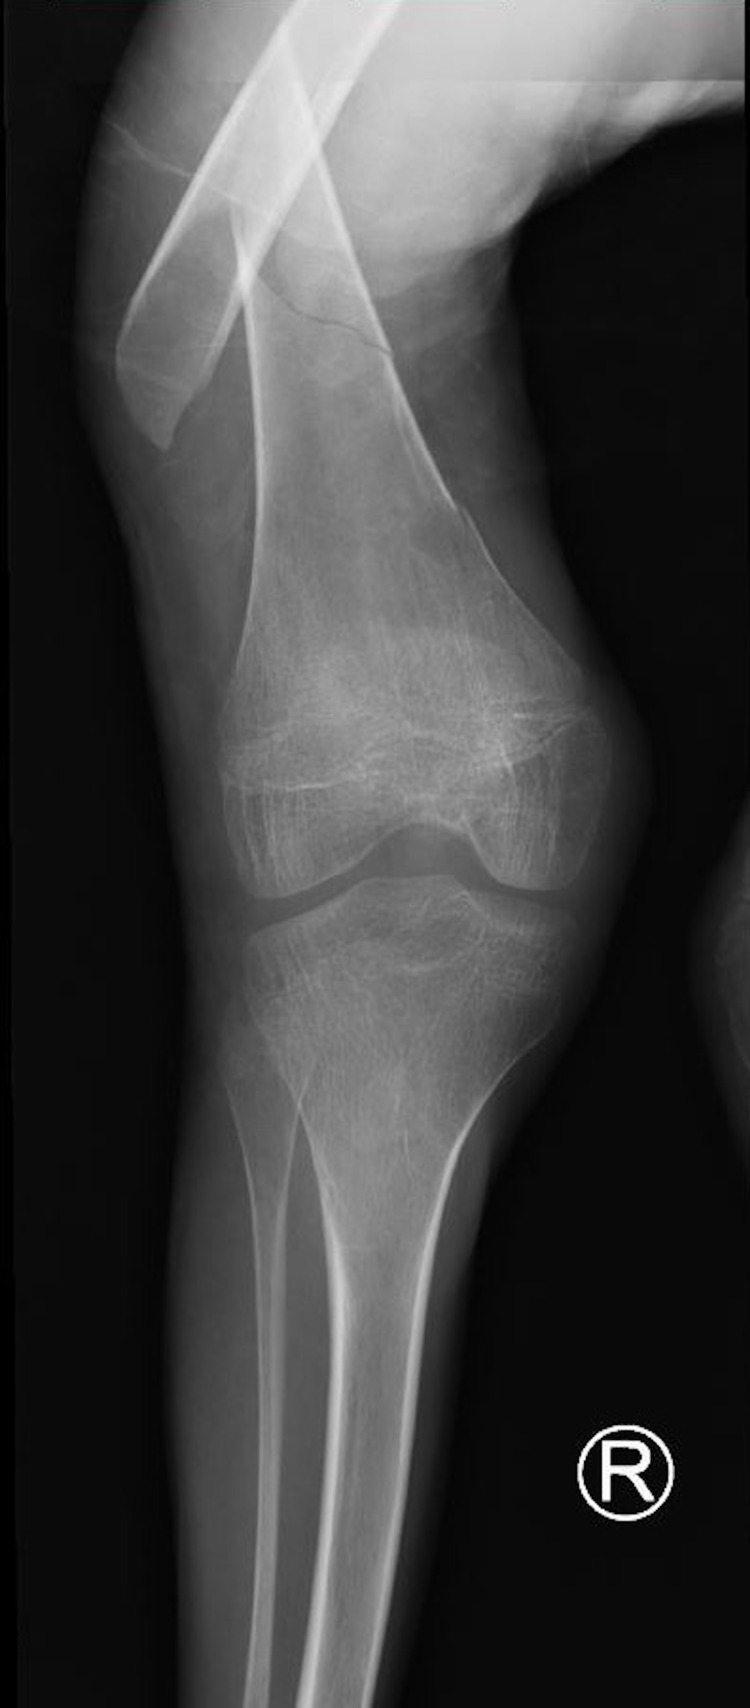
An X-ray image of the knee showing the knee joint and the distal femur physis which has not fused.
